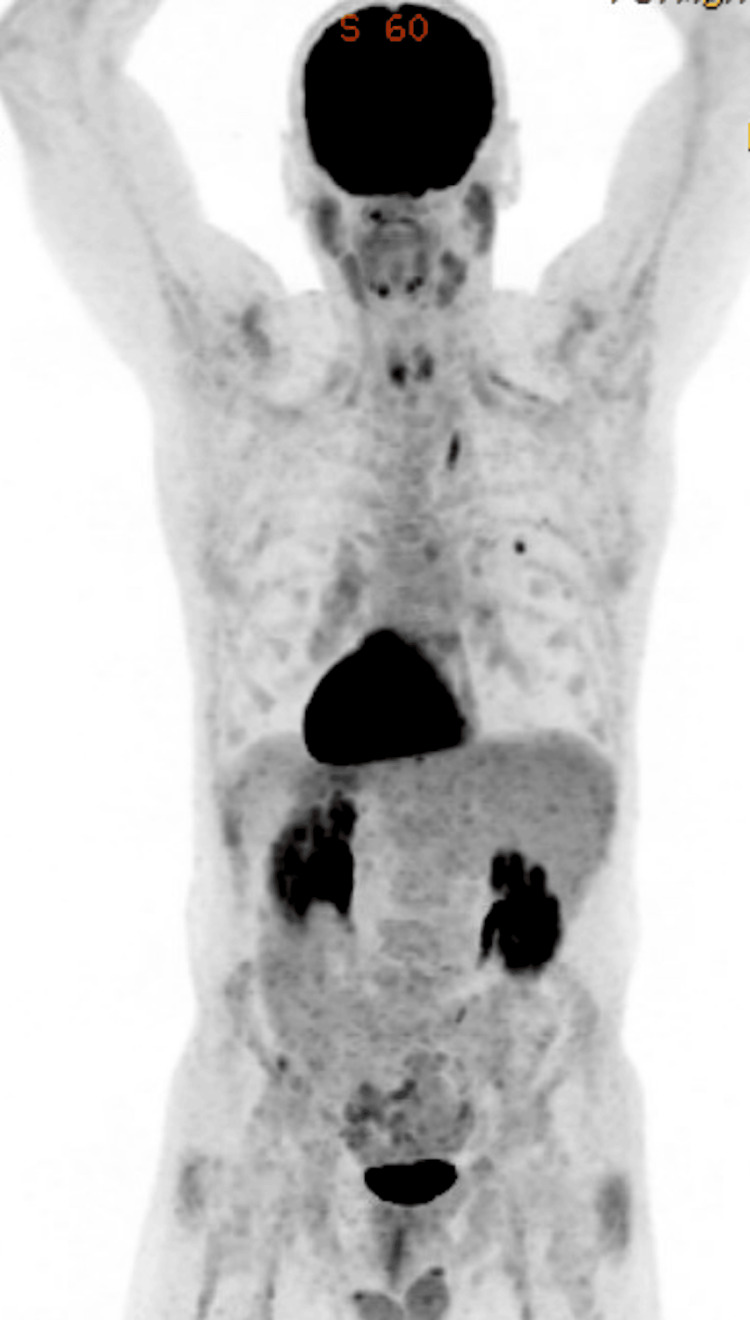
PET-CT findings after six cycles of epcoritamab and pirtobrutinib combination therapy Resolution of previously hypermetabolic left supra-and lymphadenopathy. Resolution of hypermetabolic mediastinal lymph nodes. Diminished mild peripheral uptake in the cystic left hilar node, which has decreased in size, with a maximum SUV of 2.9. Lesions are compatible with a favorable response to therapy (5PS = 3) PET-CT: positron emission tomography-computed tomography; SUV: standard uptake variable
